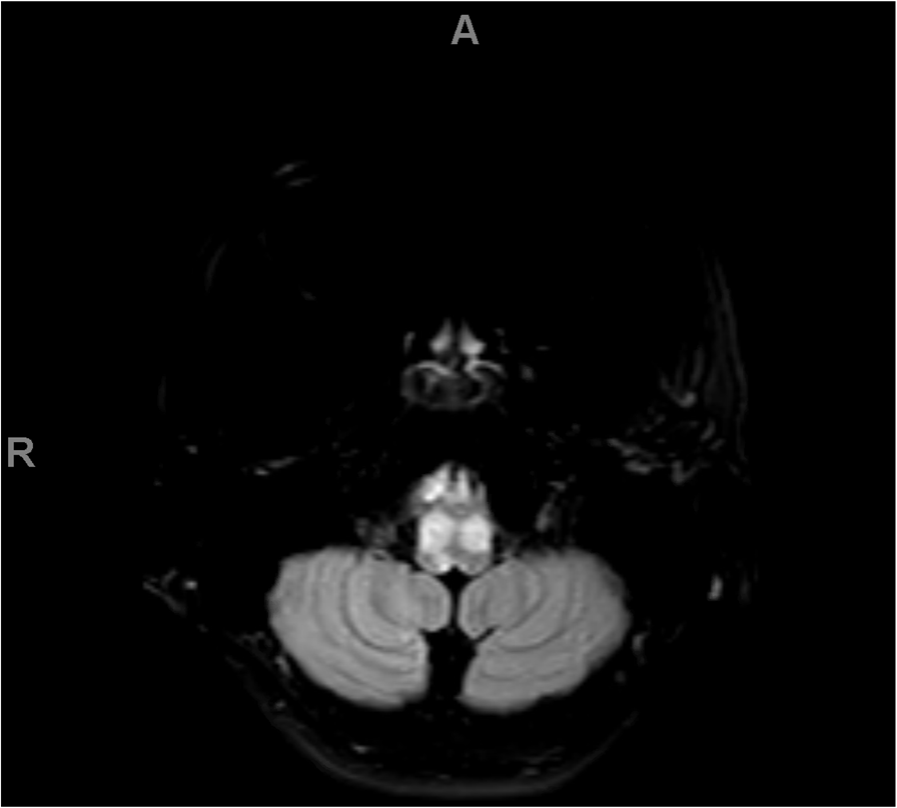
FLAIR axial images demonstrating high signal alterations in the ventral medulla
